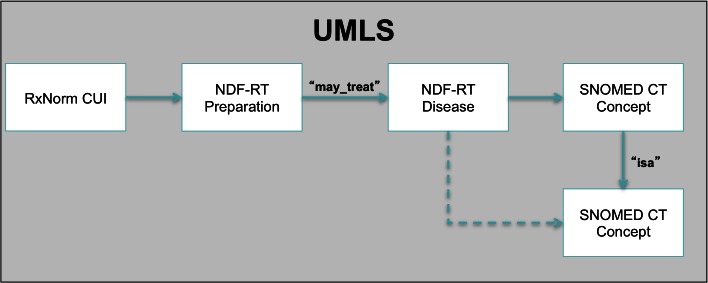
SMART RxNorm medications are mapped to SNOMED CT problems using the NDF-RT “may treat” linkage as intermediary. Adapted from [26]; used with permission.
